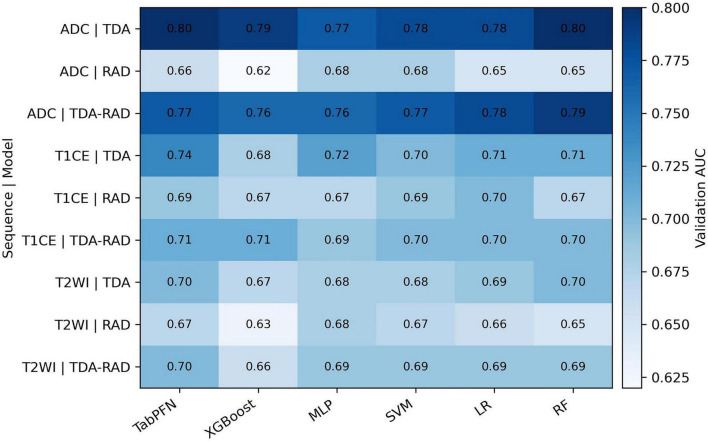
Predictive performance of TDA, RAD, and TDA-RAD models across classifiers and MRI sequences.
